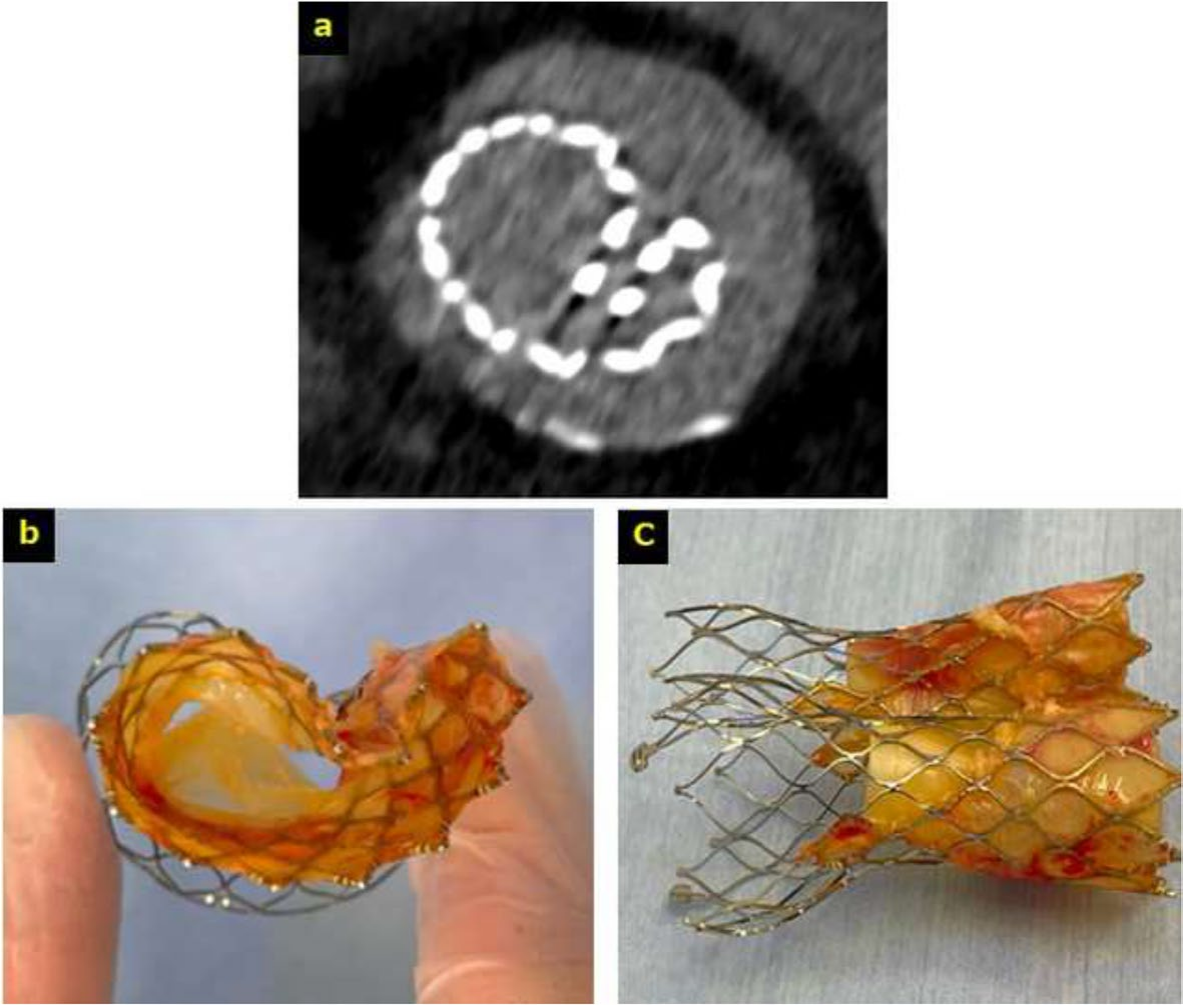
Imaging of infolding and explantation. a Postoperative computed tomography image showing that the valve is severely infolded. b, c The explanted Evolut FX valve
